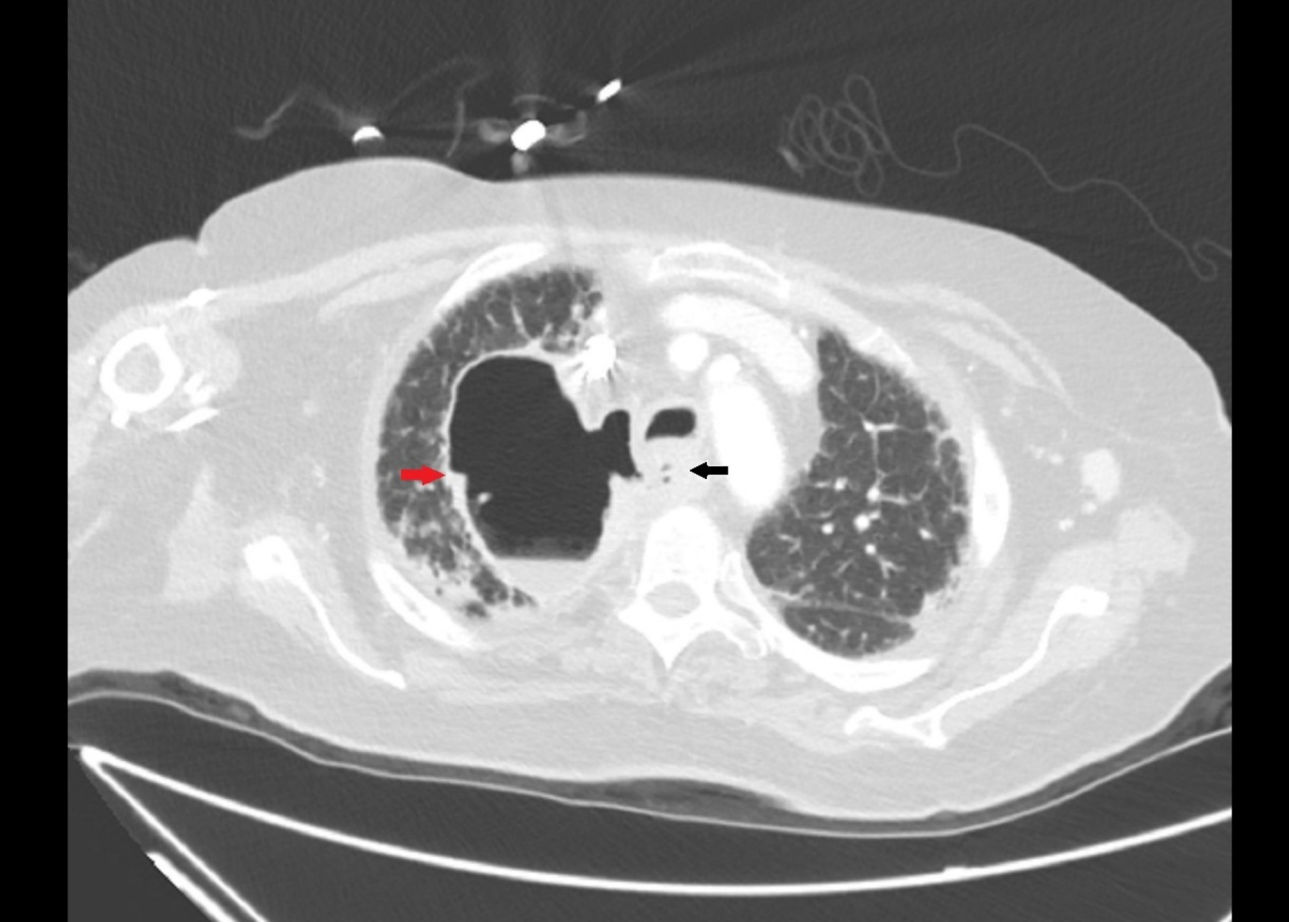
Esophageal-pulmonary fistula Computed tomography of the chest with contrast appears to show a fistulous communication with the esophagus (black arrow) along with a right upper- lobe cavitary lesion (red arrow) with irregular wall thickening.
